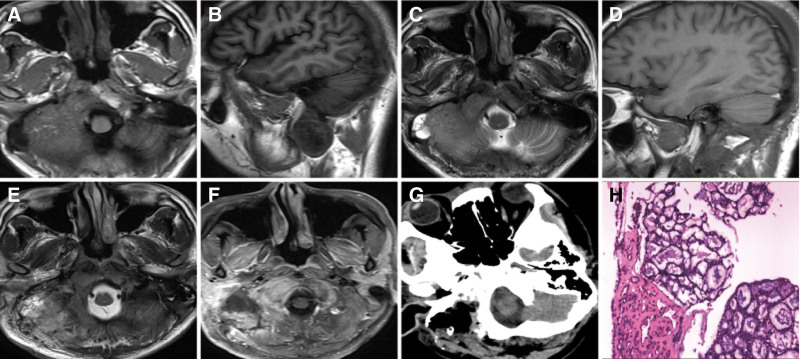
Case 1. Magnetic resonance images showing isointense T2 (A) and isointense T1 (B and C) signals on the right jugular foramen. Magnetic resonance images (D–F) and axial CT (G) revealing gross total resection. Pathological examination (H) revealed the diagnosis of ACC. H & E, original magnification = 200×. ACC = adenoid cystic carcinoma, CT = computed tomography.
